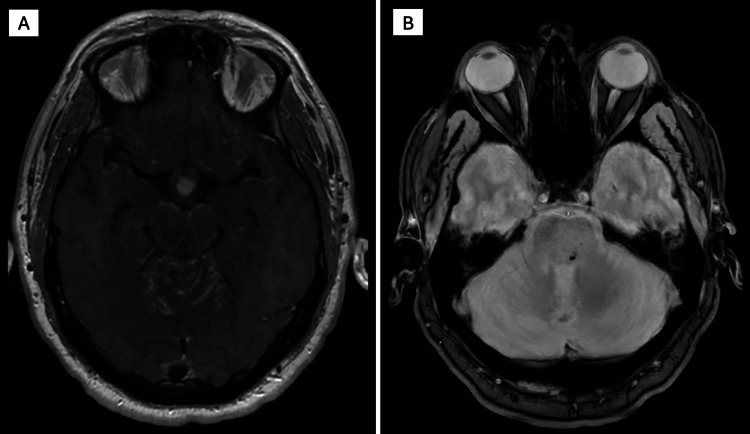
Brain MRI showing hypothalamic/pituitary enhancement and pontine microhemorrhage in neurosarcoidosis (A) Post-contrast T1 brain MRI showing leptomeningeal enhancement and thickening of the hypothalamus and proximal pituitary stalk; (B) MRI gradient echo sequences (GRE) sequence showing posterior medial pontine microhemorrhage.
